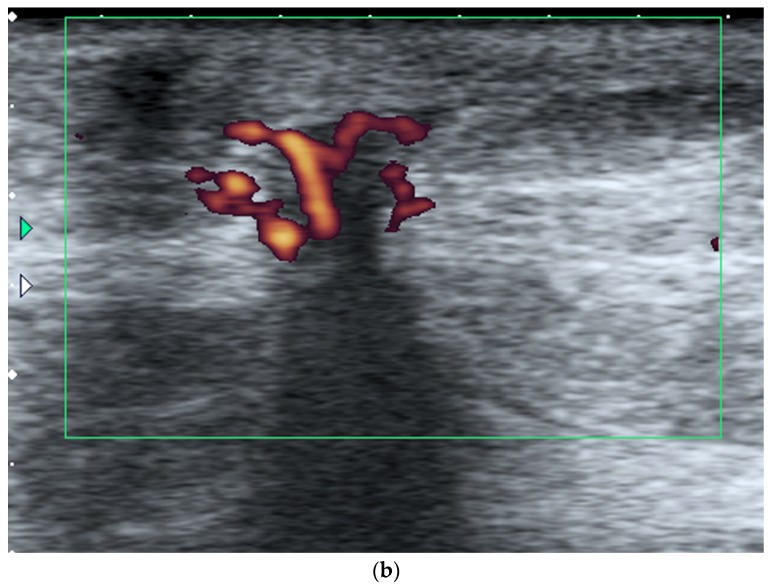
Screening routine right CC mammogram in a 60 year-old patient (a). The right CC mammogram demonstrates a small irregular spiculated mass in the retroareolar area (arrow). US images of the right breast show an (b); irregular spiculated non-parallel hypoechoic mass associated with an increased vascularity at color Doppler interrogation. Pathology yielded low-grade invasive ductal carcinoma.
